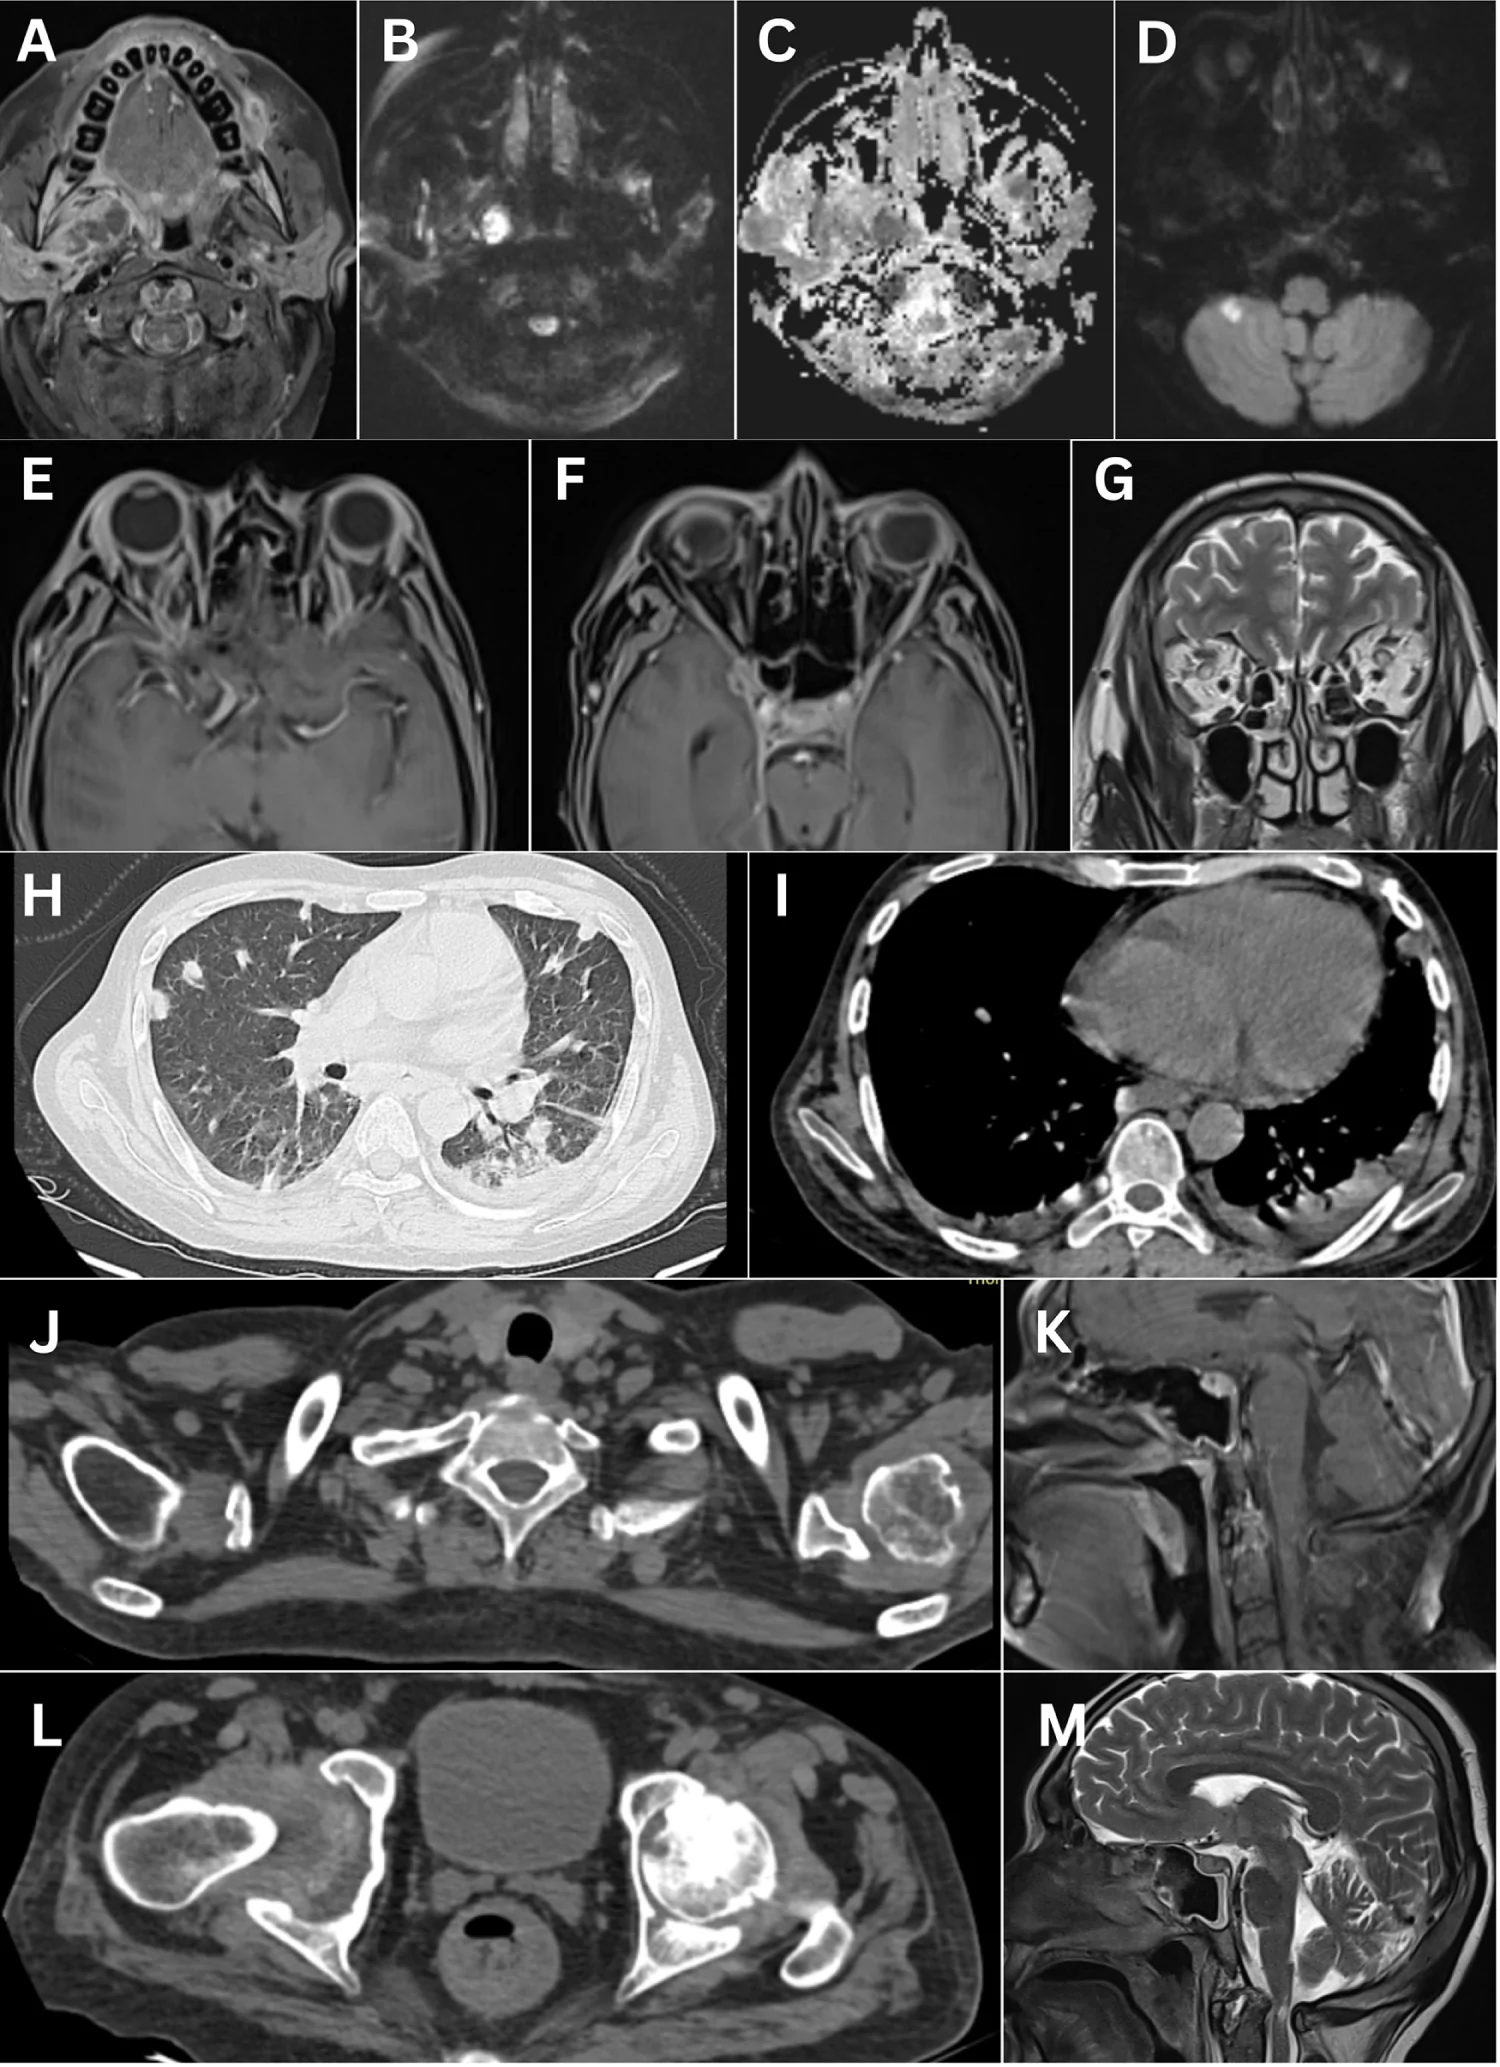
(A–G) MRI brain and orbit: septic spread and cavernous sinus involvement. (H–M) Systemic dissemination: thorax, pelvis, shoulders, and craniovertebral junction. (A) Axial T1-weighted fat-suppressed post-contrast image shows peripheral ring-enhancing collection in the right masticator space involving the pterygoid muscles, consistent with abscess. (B) Similar enhancing lesion is seen in the left maxillary space extending to the retromolar trigone, suggesting bilateral deep facial space infection. (C) Diffusion-weighted sequences demonstrate diffusion restriction in the bilateral masticator spaces, more pronounced on the right. (D) Diffusion-weighted imaging with corresponding ADC map shows focal diffusion restriction in the right anterior cerebellar hemisphere, compatible with abscess. (E) Axial post-contrast T1-weighted fat-suppressed images reveal intraluminal filling defects within both superior ophthalmic veins, consistent with superior ophthalmic vein thrombosis. (F) Post-contrast images demonstrate abnormal thickening and enhancement of the cavernous sinus, compatible with cavernous sinus thrombosis. (G) Coronal T2-weighted orbital sequences show dilated superior ophthalmic veins with loss of normal flow voids and hyperintense signal, indicating orbital venous congestion. (H) CT thorax (lung window) shows multiple soft-tissue density nodules, several with central cavitation, suggestive of septic emboli. (I) CT thorax (soft tissue window) reveals bilateral pleural effusions. (J) Non-contrast CT pelvis demonstrates fluid collections in both hip joints (right > left) with articular surface irregularities. (K) Axial T1-weighted fat-suppressed MRI shows abnormal enhancement at the craniovertebral junction. (L) Similar inflammatory fluid and synovial changes are seen in both shoulder joints. (M) Sagittal T2-weighted MRI shows fluid extending across the craniovertebral junction with foramen magnum narrowing, spinal cord compression, and cord edema.
